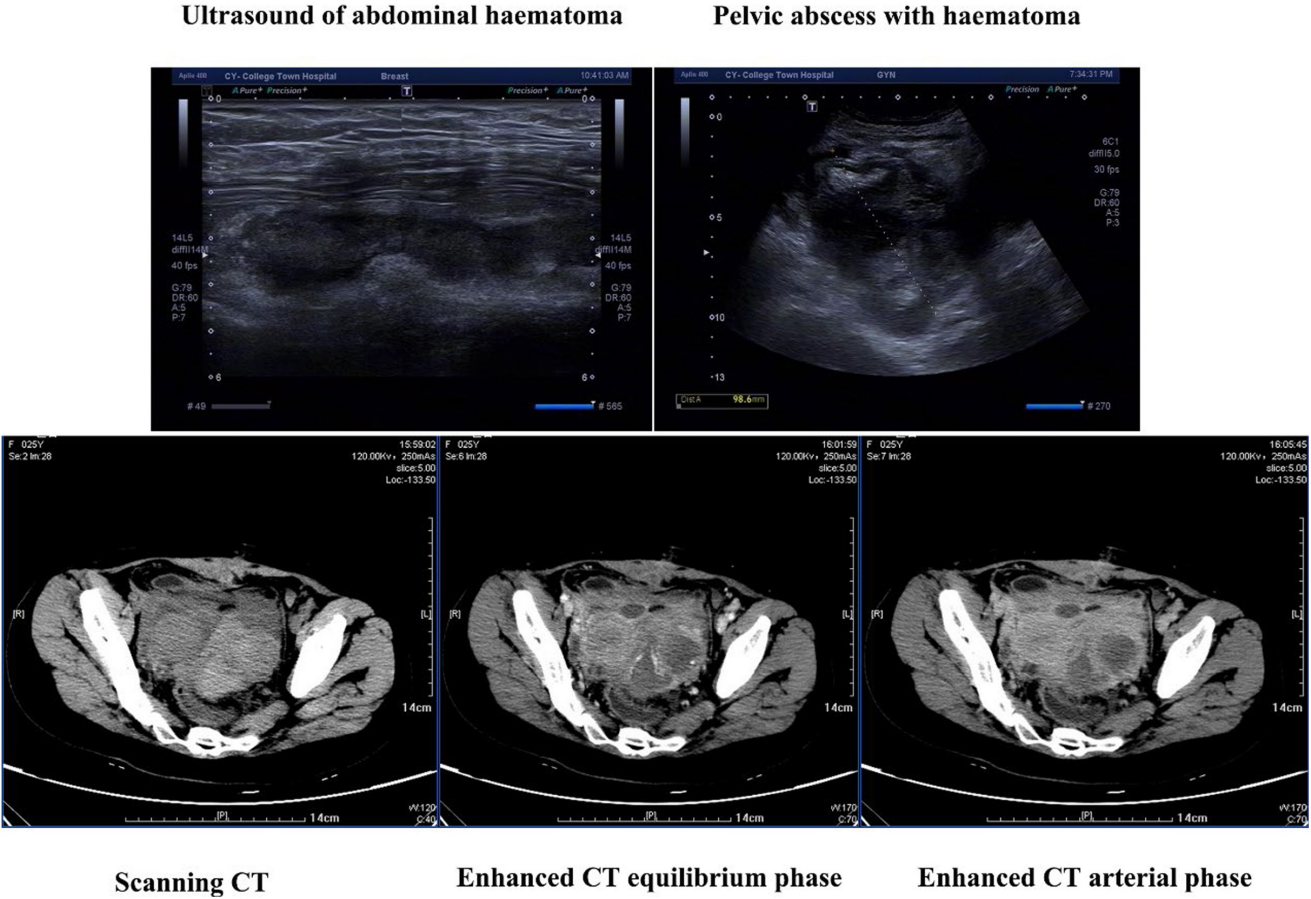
Pelvic ultrasound (abdominal wall haematoma, pelvic haematoma with abscess) and CT images (pelvic haematoma with abscess).
